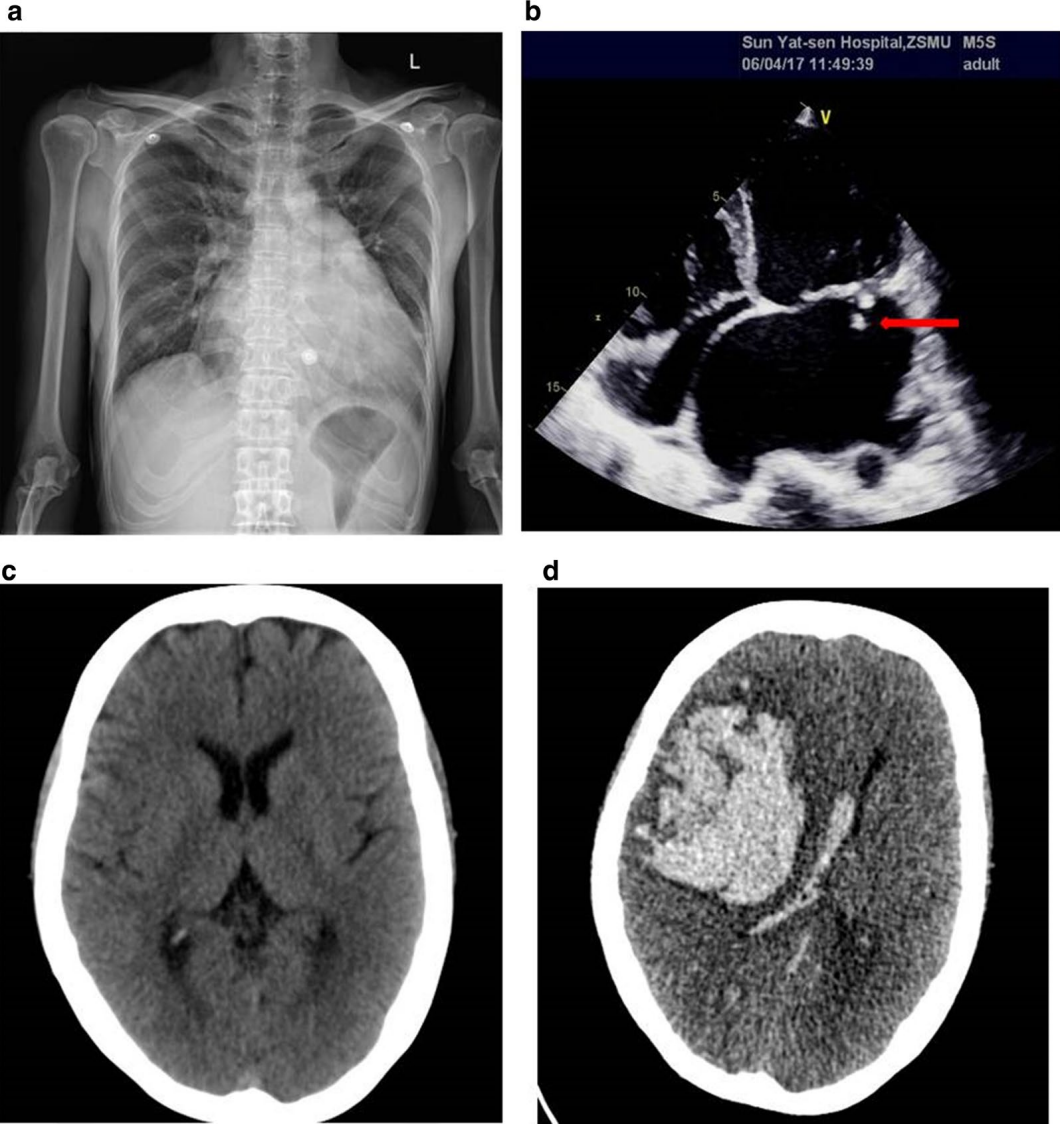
Image examination of the patient. a The chest X-ray plain film showed cardiac image enlargement in left atrium, right ventricle and left ventricle, together with bilateral pulmonary congestion. b Echocardiography examination showed mitral valve prolapse and multiple vegetations (the largest one was about 14 mm, arrow). c Computerized tomography scan of the brain showed mild ischemia at bilateral basal ganglia. d Computerized tomography scan of the brain showed massive intracerebral hemorrhage in the right frontal–temporal lobe and lateral ventricle
